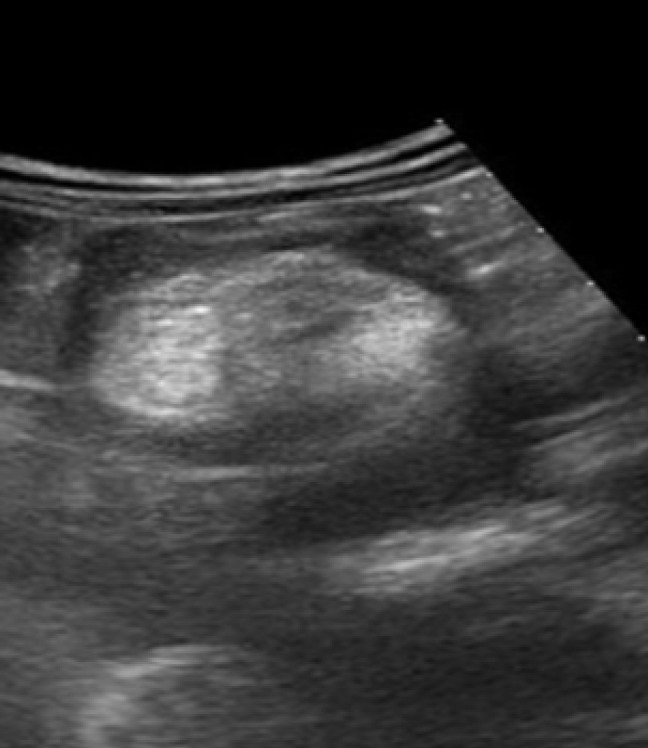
An Ultrasound Picture Showing a Mixed Echogenic (Hyperechoic with Relative Acoustic Shadowing and Hypoechoic) Mass
